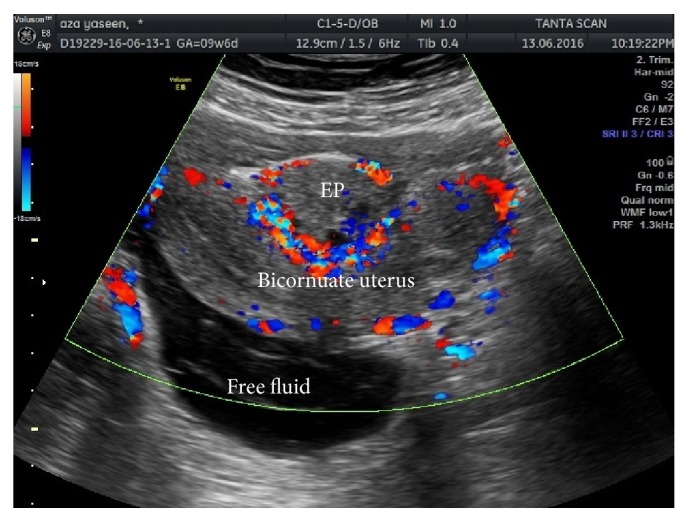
Doppler ultrasound showing bicornuate uterus and pelvic free fluid with the ectopic pregnancy site showing “Ring of Fire.” EP: ectopic pregnancy.
